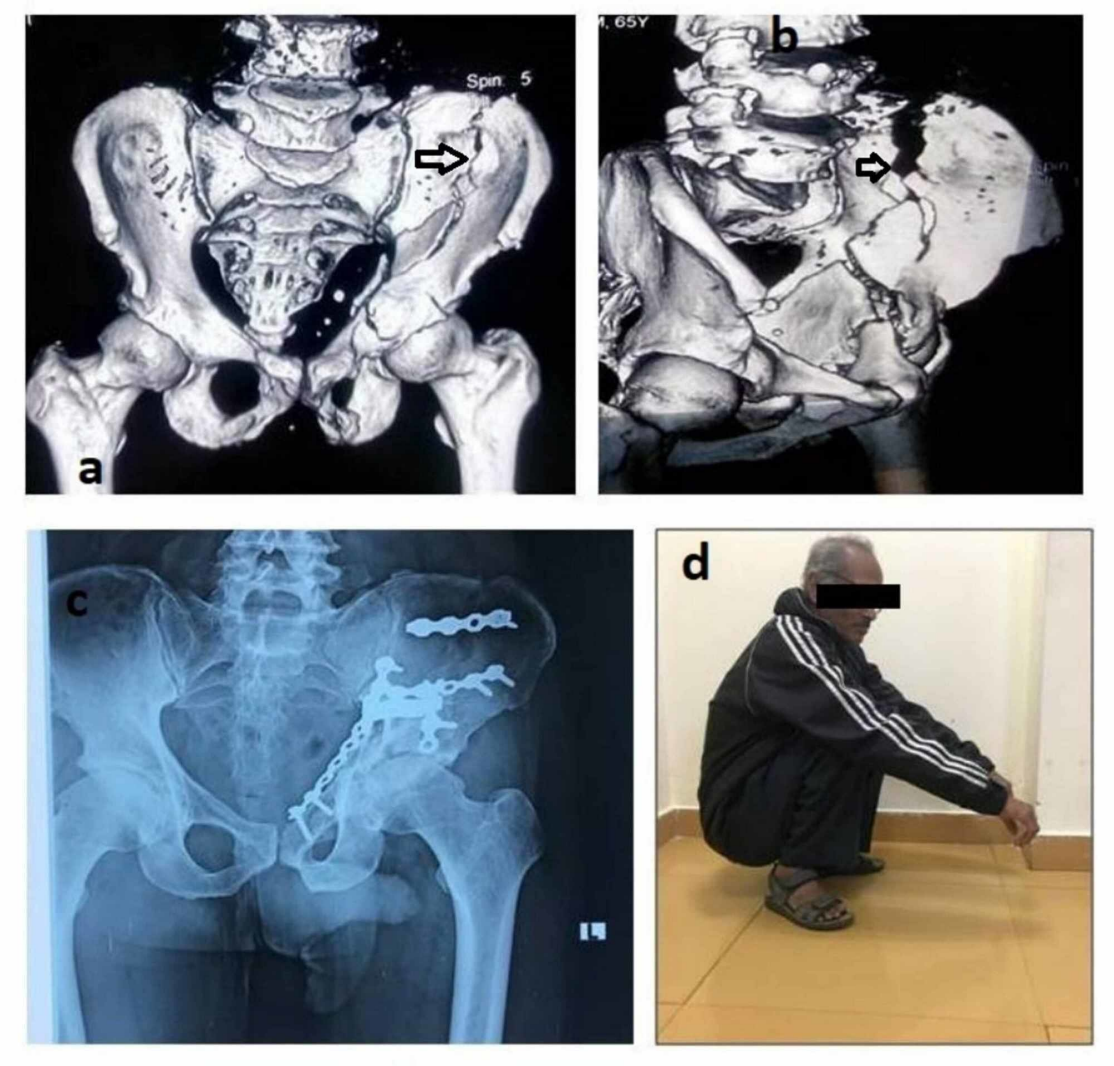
A case of anterior column fracture extending high in iliac blade (arrows) with final postoperative X-rays and functional outcome a, b: preoperative CT; c: postoperative radiograph; d: clinical photograph of the patient squatting CT: computed tomography
